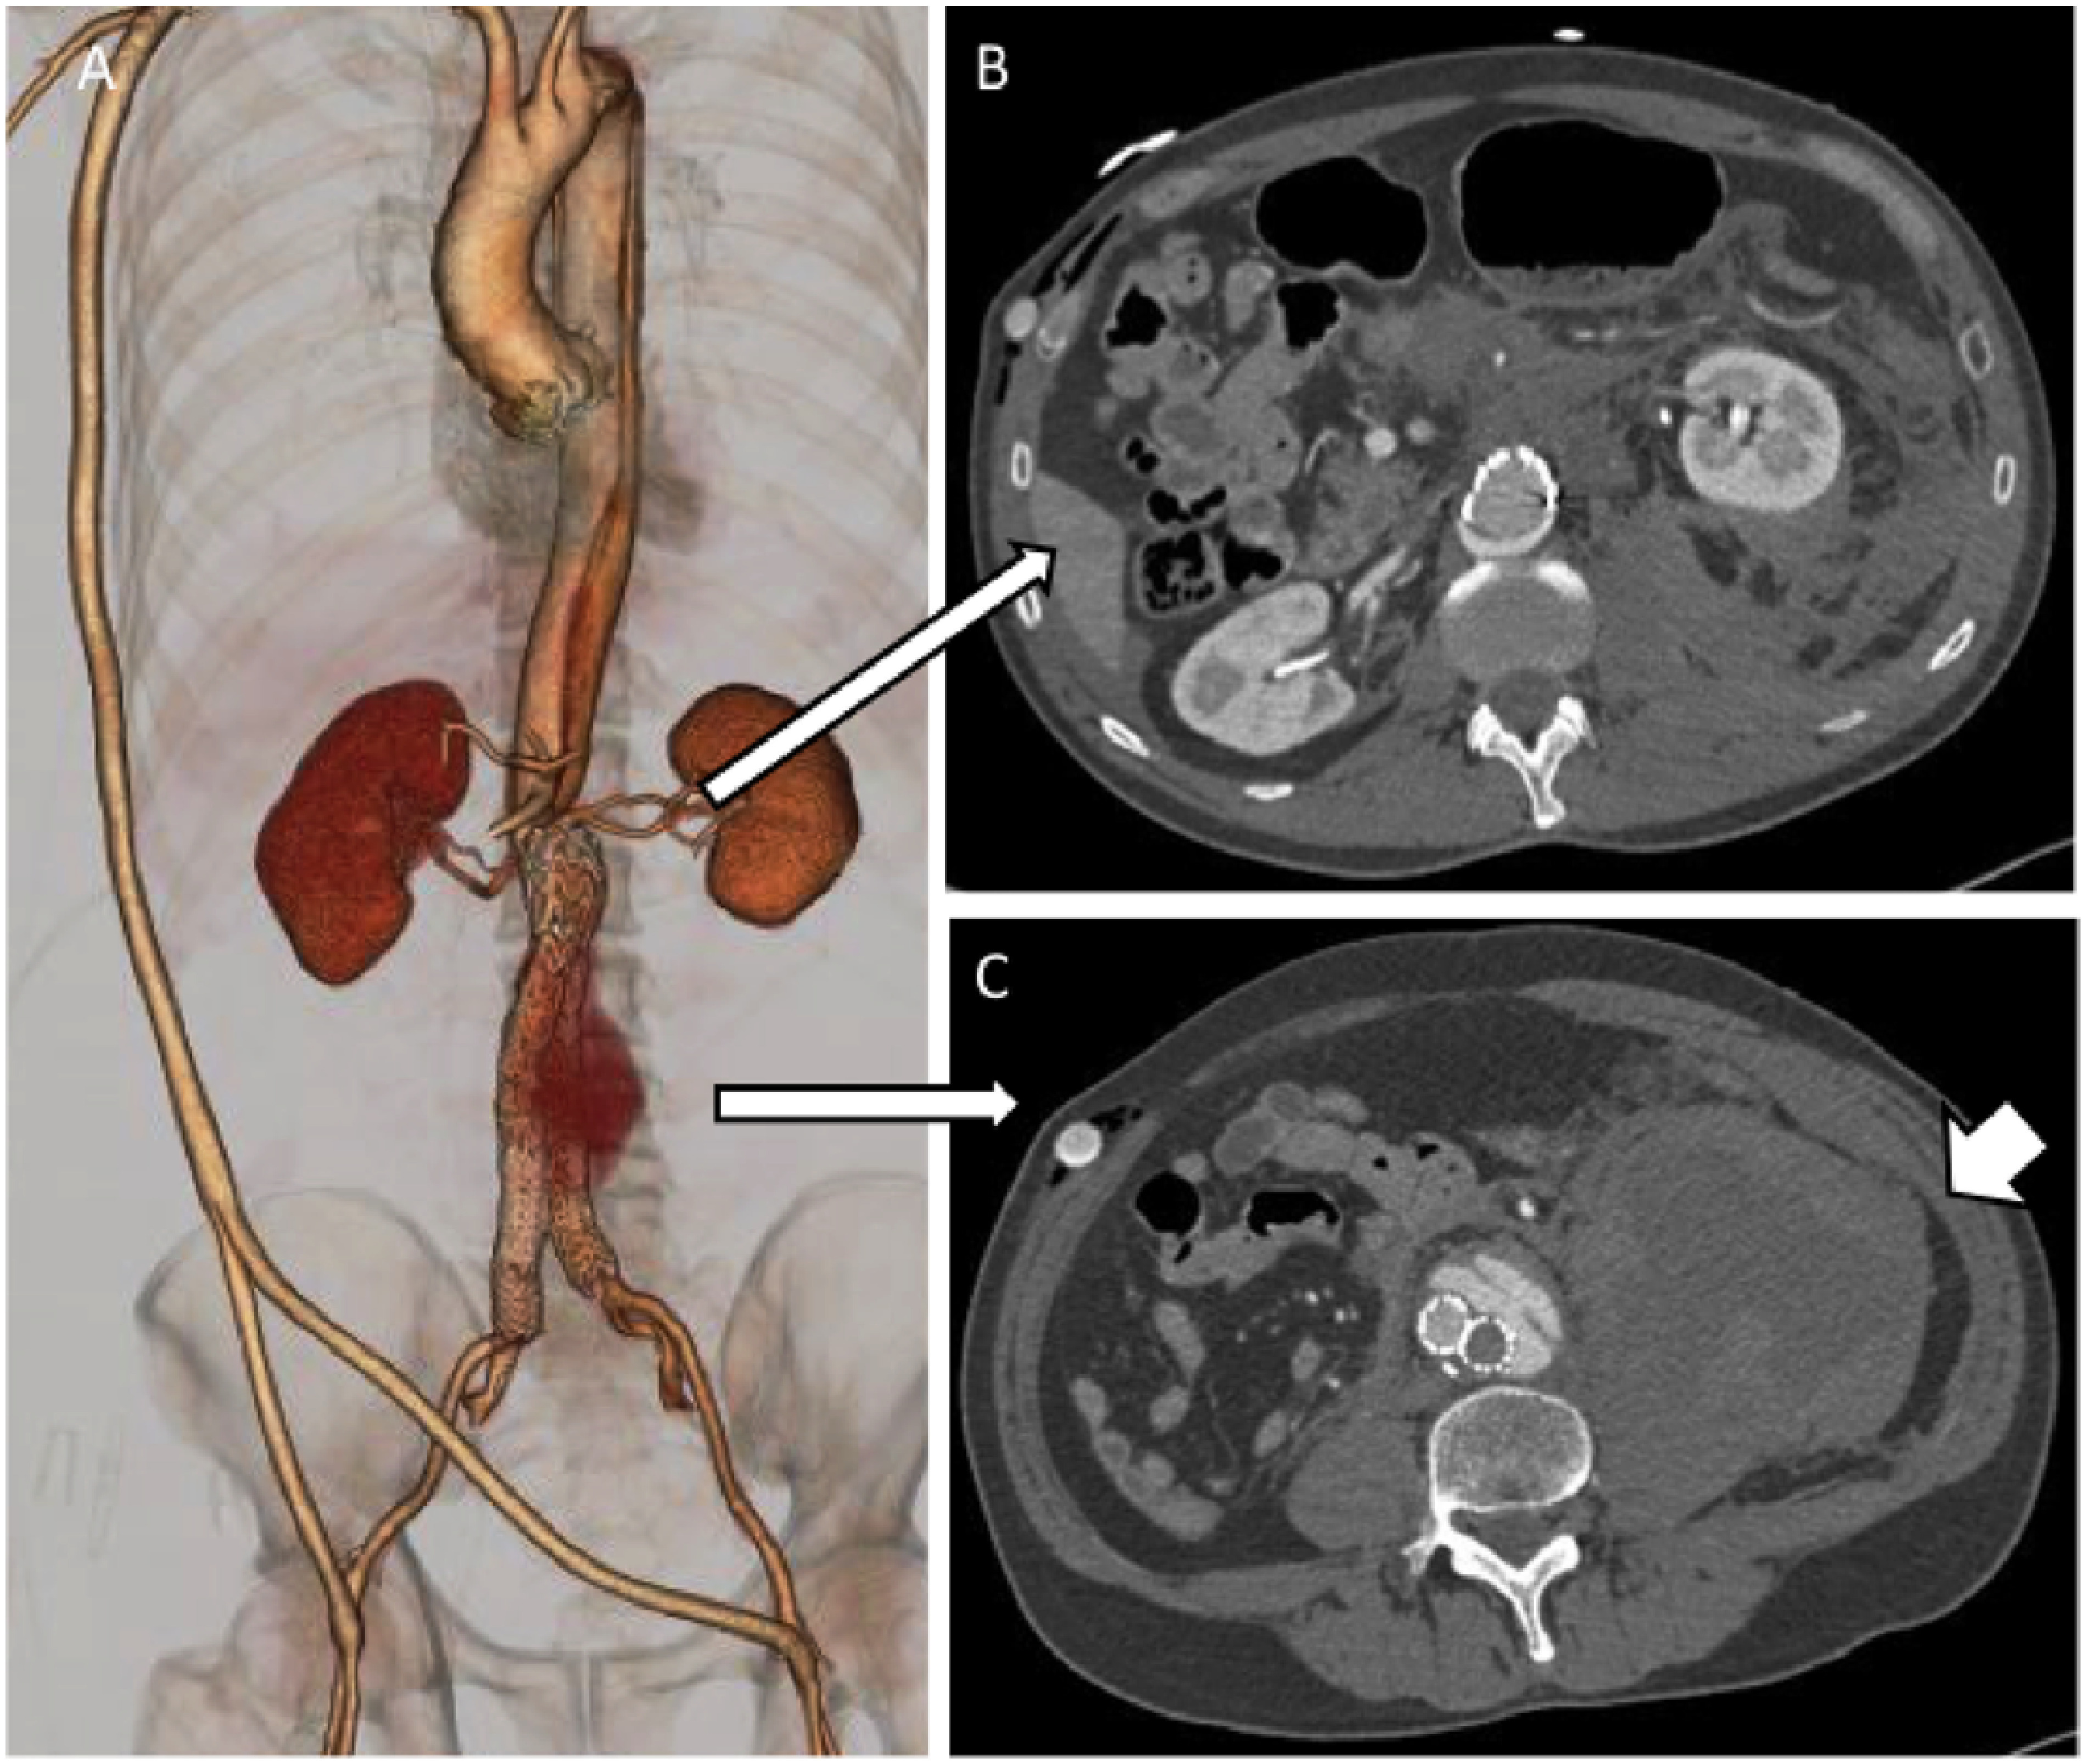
Fig. 3 (A), (B) Contrast-enhanced CT showed that the stent graft was re-expanded by the retrograde blood flow. (C) It was revealed that the dissecting abdominal aortic aneurysm has ruptured after the right axillo-bifemoral bypass operation. A large amount of hematoma was observed in the left retroperitoneum at the position of terminal aorta.
